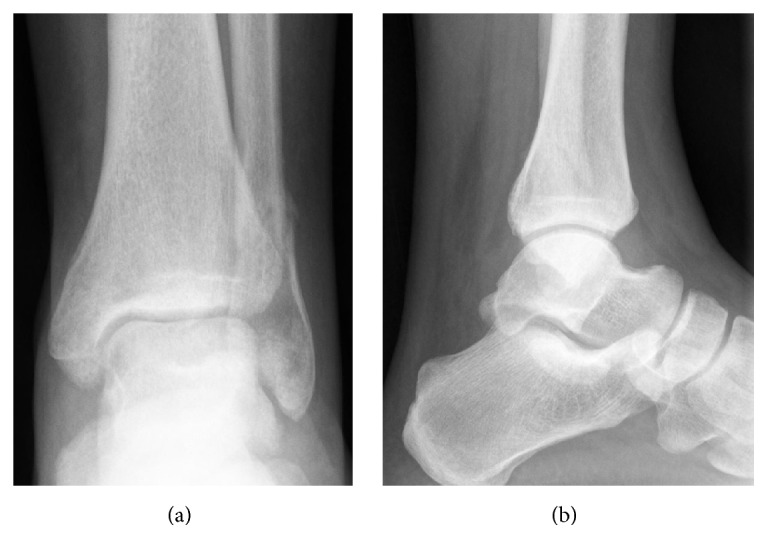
Plain (a) anteroposterior and (b) lateral radiographs of the left ankle at four weeks following injury, showing good alignment with callus formation at the fracture site.
